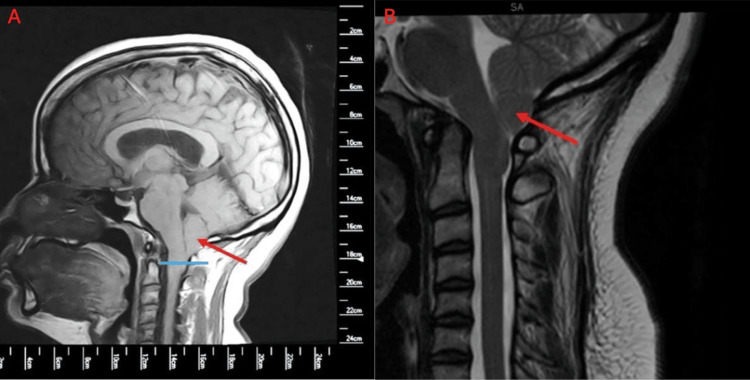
Pre-operative sagittal T1-weighted MRI A. 12-mm cerebellar tonsillar ectopia below McRae line (red arrow, blue line). B. Craniocervical junction crowding with obliterated cerebrospinal (CSF) spaces (red line)
